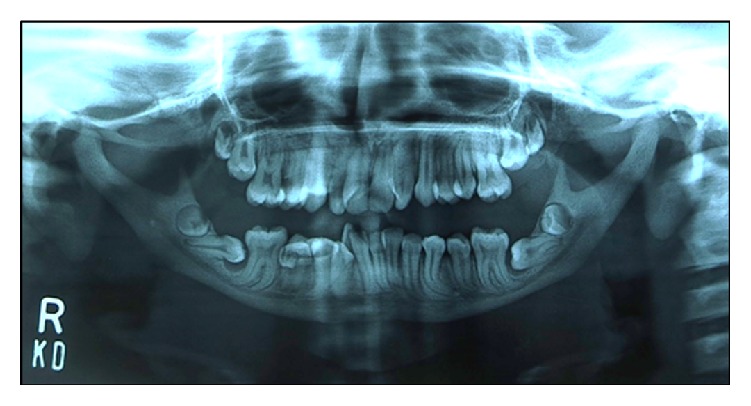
Panoramic radiographic findings.
